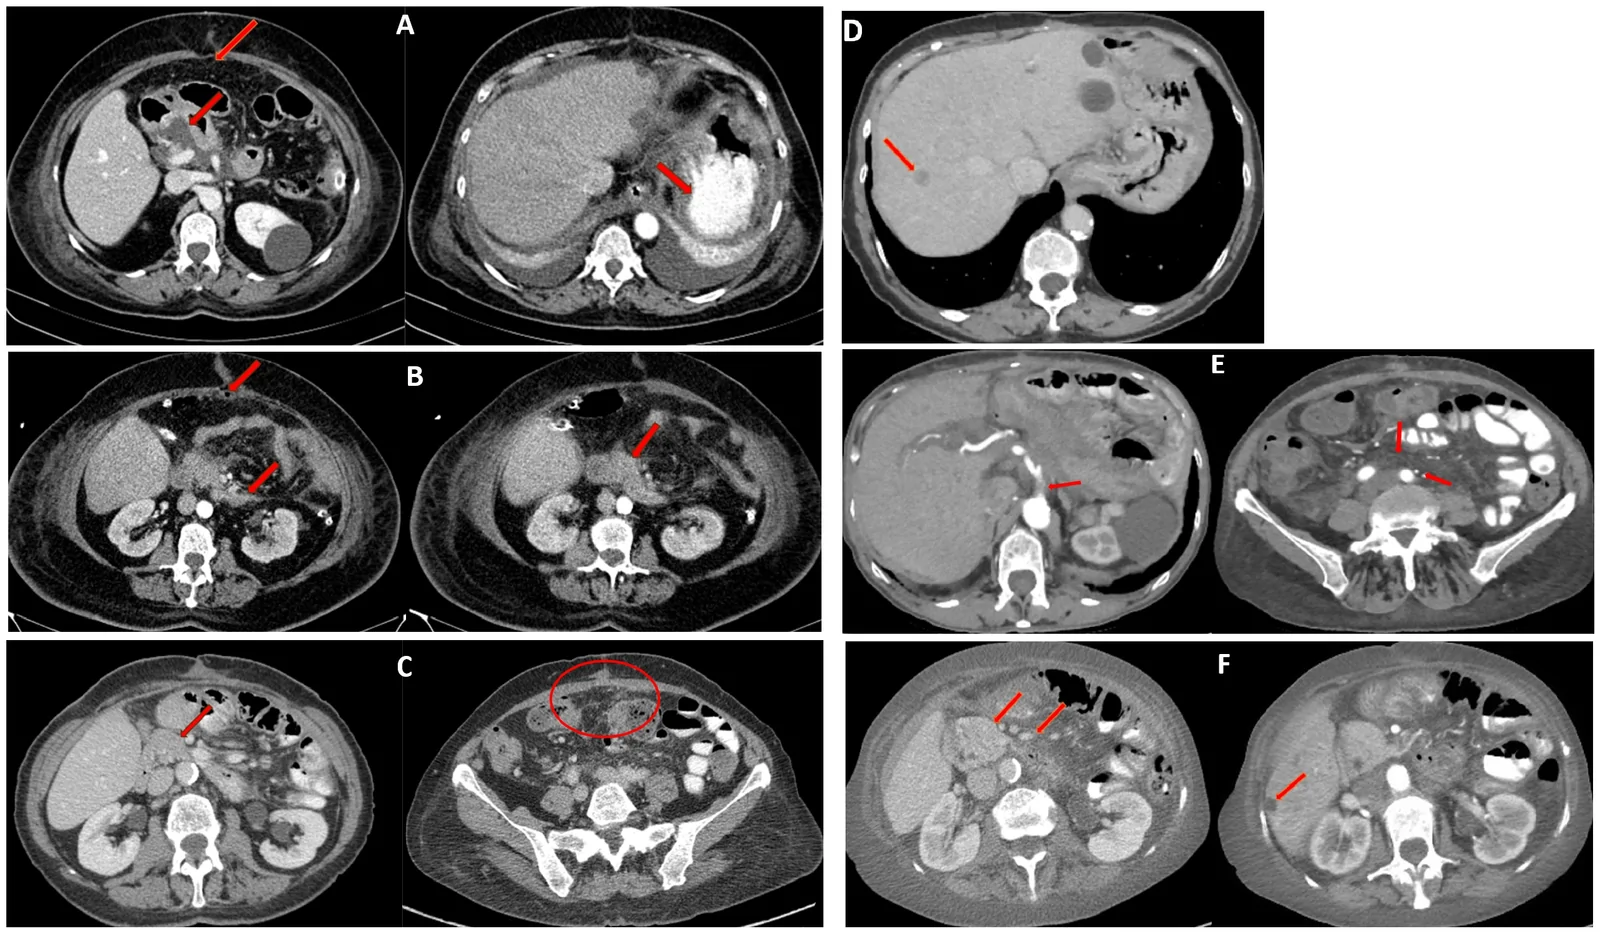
Follow-up CT scans: (A) a fistula located on the posterior gastric wall with intraperitoneal extravasation of the oral contrast, intraperitoneal air bubbles, and parafluid collection adjacent to the pancreatectomy site, in the mesogastric and left subphrenic regions; (B) no evidence of the previously mentioned small air bubbles, except for one at the level of the laparotomy incision; (C) and (D) progressive disease: mesenteric and peritoneal infiltration, with adenopathy and peritoneal nodules; (E) the tumor involved the superior mesenteric vein, superior mesenteric artery, and celiac trunk; (F) dimensional progression of the nodular hepatic lesion and new peritoneal nodules. Red arrows and circles are used to pinpoint and highlight the specific CT anomalies.
